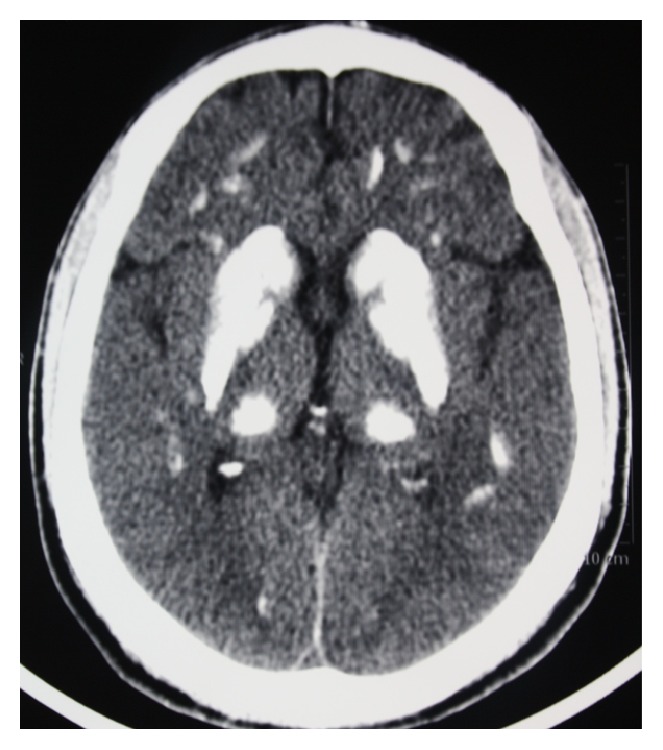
Bilateral symmetrical calcifications on cranial CT.
